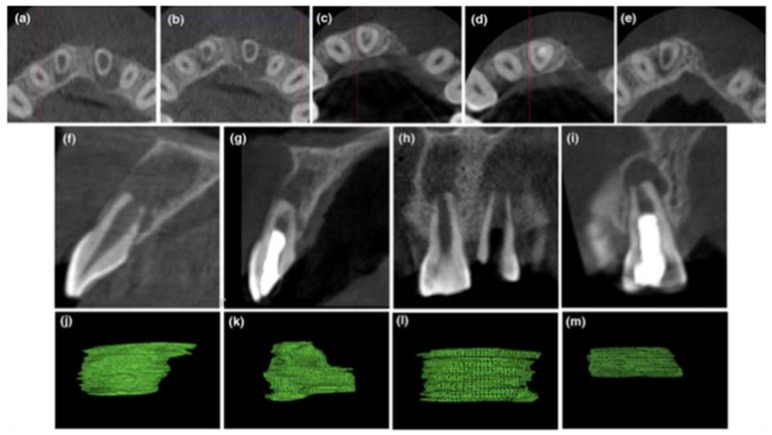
CBCT images of preoperative and three-year follow up of REP in 11. (a,b) pre-operative axial view revealing root canal dentin thickness and periapical radiolucency at midroot level (a) and apical section (b); (c–e) post-operative axial view in the middle and apical region revealing a reduction in size of periapical radiolucency, thickening of root canal wall and mineralized layer above the Biodentine (e); (f,g) pre-operative and post-operative sagittal view showing a reduction in periapical radiolucency; (h,i) pre-operative and post-operative coronal view showing a reduction in periapical radiolucency and a thickening of the root canal wall; (j,k) pre-operative and post-operative axial images showing a reduction in volume of periapical lesion by 86% (measured using Volux—Horos viewer for Mac (V2.0.2); (l,m) pre-operative and post-operative sagittal images showing a reduction in volume of periapical lesion by 78% (measured using Volux—Horos viewer for Mac (V2.0.2).
